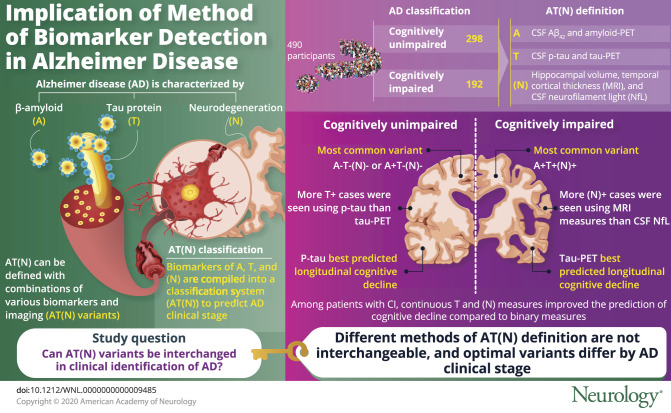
Alzheimer disease (AD) is characterized by accumulation of β-amyloid (Aβ) and tau, atrophy, and cognitive decline.^1^ The different brain changes can be monitored by CSF biomarkers, MRI, and PET. The National Institute on Aging and Alzheimer's Association has proposed a framework for research^2^ based on the idea that biological processes start before symptoms in AD. The framework suggests that biomarkers of Aβ (A), tau (T), and neurodegeneration (N) can be compiled into a classification system (AT[N]). The framework is purposely agnostic to the details of operationalization, and it is unknown how different variants of AT(N) (using different biomarkers) compare. We therefore tested a variety of AT(N) approaches, with different combinations of biomarkers, which were compared in terms of prevalence in cognitively unimpaired (CU) and cognitively impaired (CI) participants and to predict cognitive decline. We hypothesized that different AT(N) variants would have considerable differences in category prevalence and that the prediction of longitudinal cognitive decline would vary both by AT(N) variant and predictor data type (binary vs continuous).
